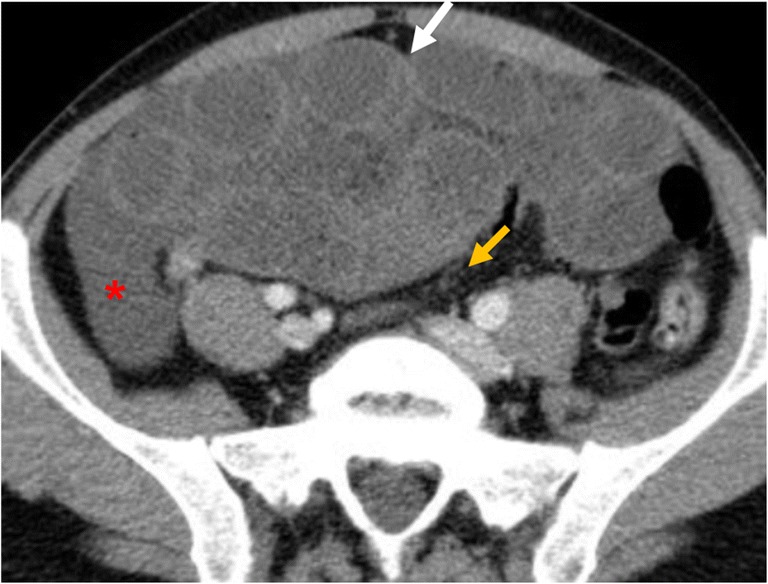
Gasless abdomen. Contrast-enhanced CT image of the abdomen in a 62-year-old female with small bowel wall ischaemia due to venoocclusive disease. Contrast-enhanced CT image of the abdomen shows dilated and mainly fluid-filled bowel loops and gasless abdomen (white arrow). Ascites (*) and vascular engorgement (orange arrow) are also seen
